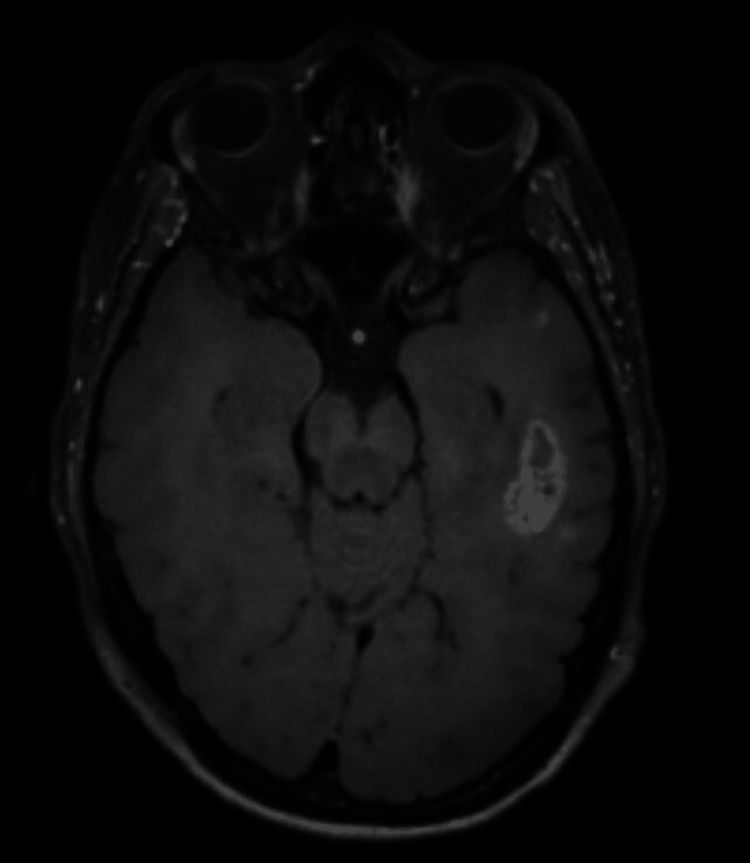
MRI cerebrum T1 sequence with contrast showing left temporal lobe brain metastasis, five weeks after start of chemoimmunotherapy with evident regression of left temporal brain metastasis.
